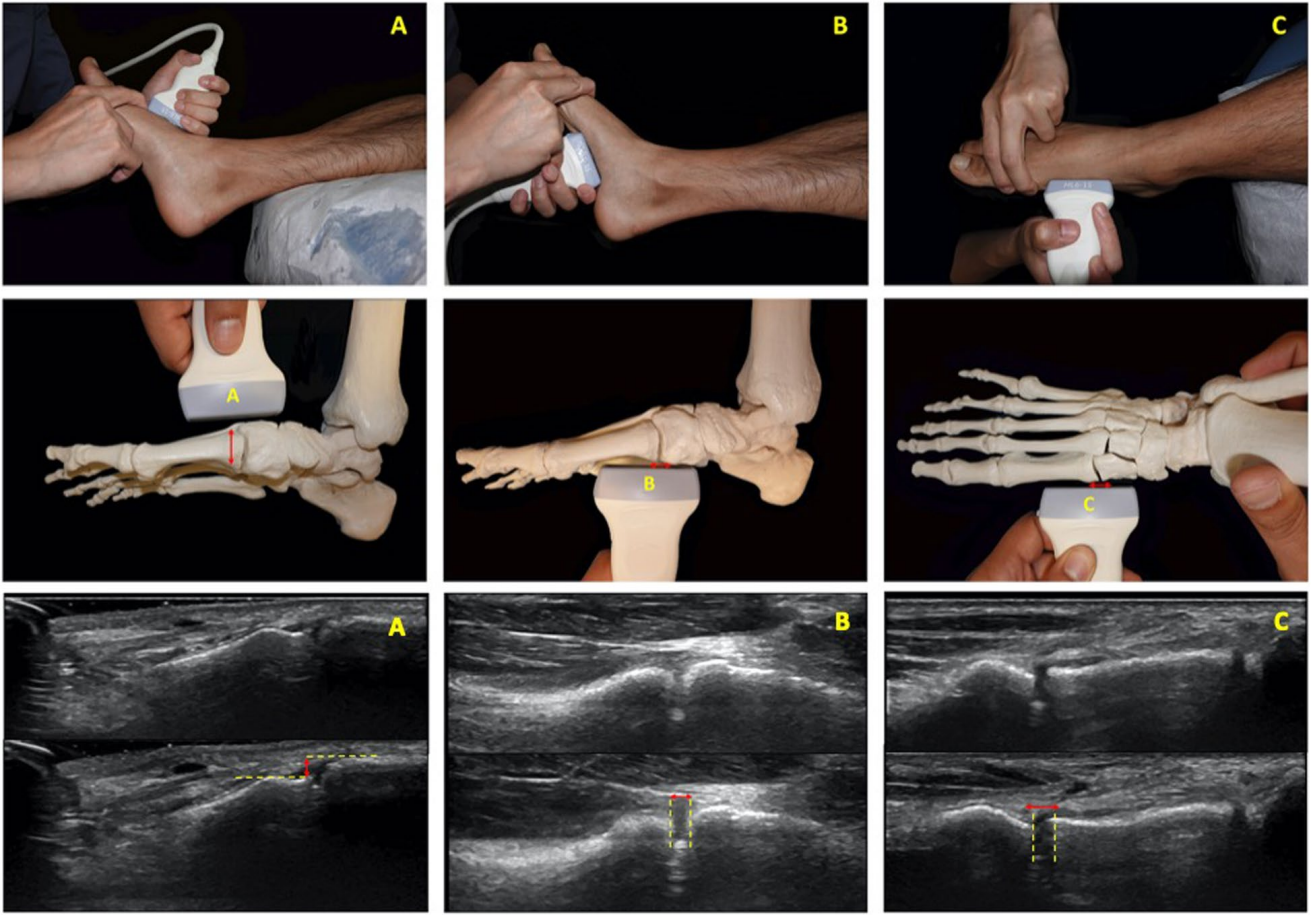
Ultrasound probe orientation and measurement. A probe placed perpendicular to the dorsum of the first metatarsocuneiform joint measures dorsal translation, representing vertical mobility (A). A probe placed perpendicular to the plantar side of the first metatarsocuneiform joint measures plantar gapping, representing mobility under weight-bearing (B). A probe placed perpendicular to the medial side of the first metatarsocuneiform joint measures medial gapping, representing horizontal mobility (C). The techniques of ultrasound measurements before and after sufficient force were applied to reach the soft tissue endpoints and no further bone movements. The distances before and after the application of force were recorded for dorsal translation (A), plantar gapping (B), and medial gapping (C)
